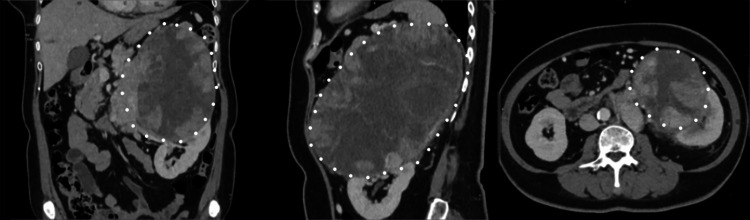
Contrast-enhanced whole abdominal CT scan Encircled in white: suprarenal mass
